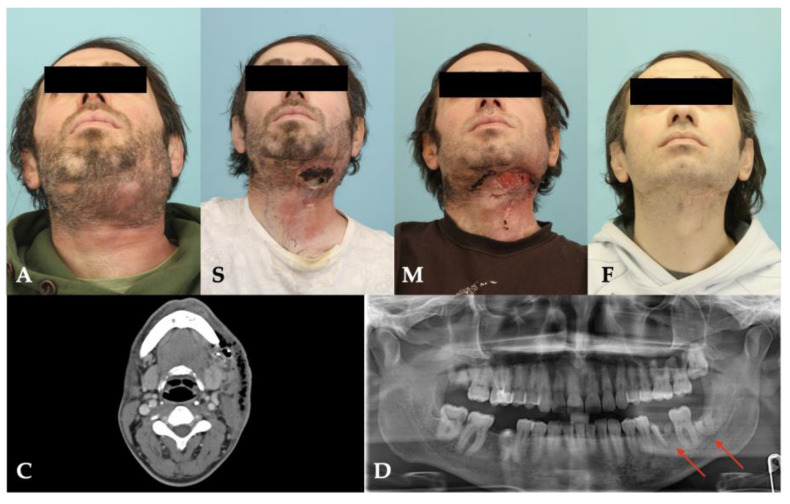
Clinical course and radiographs of patient No. 4: A: admission; S: surgery; M: maximal extent; F: final result; C: CT scan; D: dental focus (tooth remnants 36 and 38).
